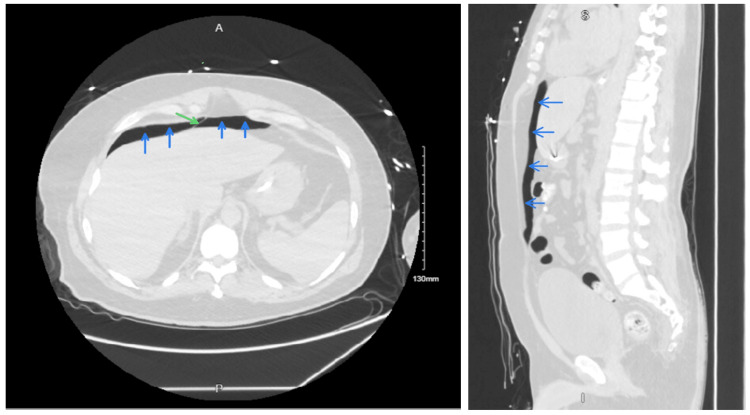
CT abdomen showing pneumoperitoneum Air in the peritoneum can be seen as a dark space (marked by blue arrows). The falciform ligament can be seen; the falciform ligament sign is marked in green. The CT abdomen is viewed in the lung window to make the pneumoperitoneum prominent.
